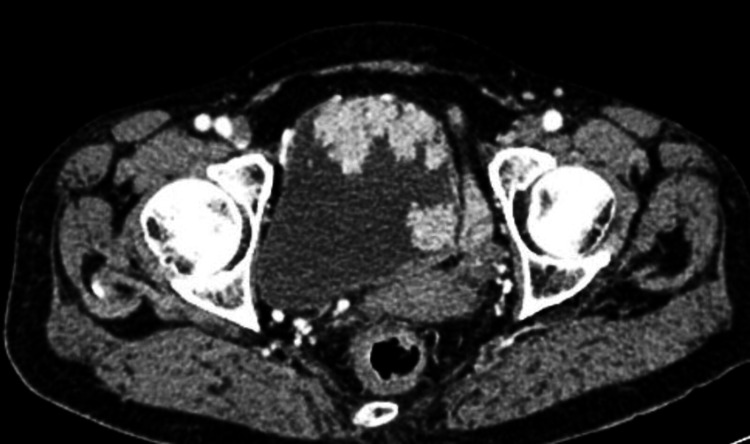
Multiple bladder masses Axial contrast-enhanced computed tomographic image showing multiple enhancing bladder masses
